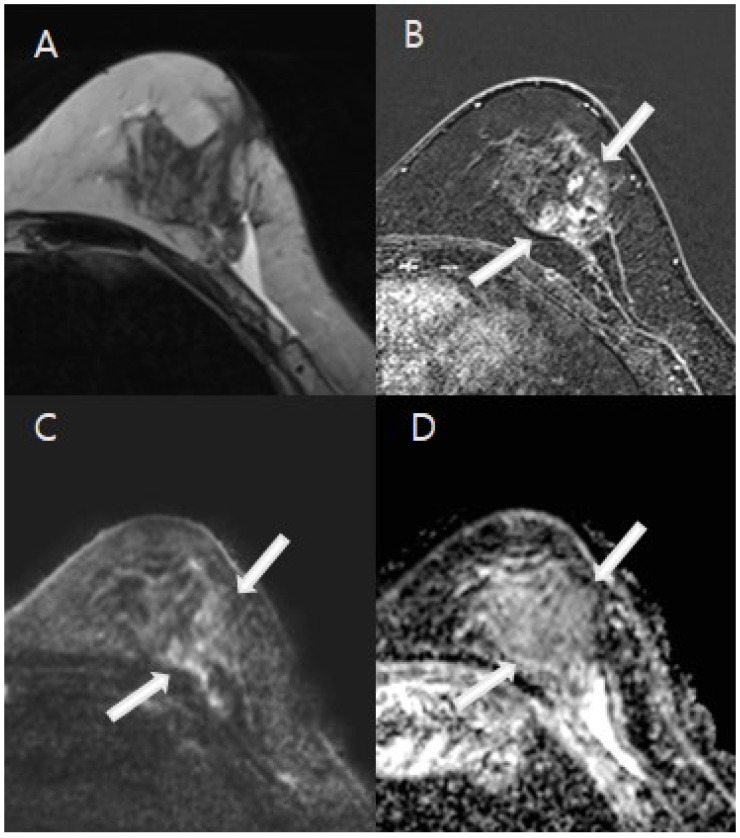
A 44-year-old woman with excisional biopsy-proven ductal carcinoma in situ (DCIS). (A). MRI demonstrates a parenchymal defect with T2 high signal intensity, suggesting fluid collection at the biopsy site in the mid outer aspect of the left breast. (B). A 2.8 cm area of regional nodular non-mass enhancement (white arrows) was noted in the medial aspect of the biopsy cavity. (C,D). Diffusion-weighted imaging (DWI) at b = 1000 mm^2^/s and the ADC map (b = 0 and 1000 mm^2^/s) indicated restricted diffusion in the corresponding area, with an ADC value of 1.3 × 10^−3^ mm^2^/s, indicative of residual malignancy (white arrows). The patient underwent a mastectomy, and final pathology confirmed a 3 cm area of DCIS.
